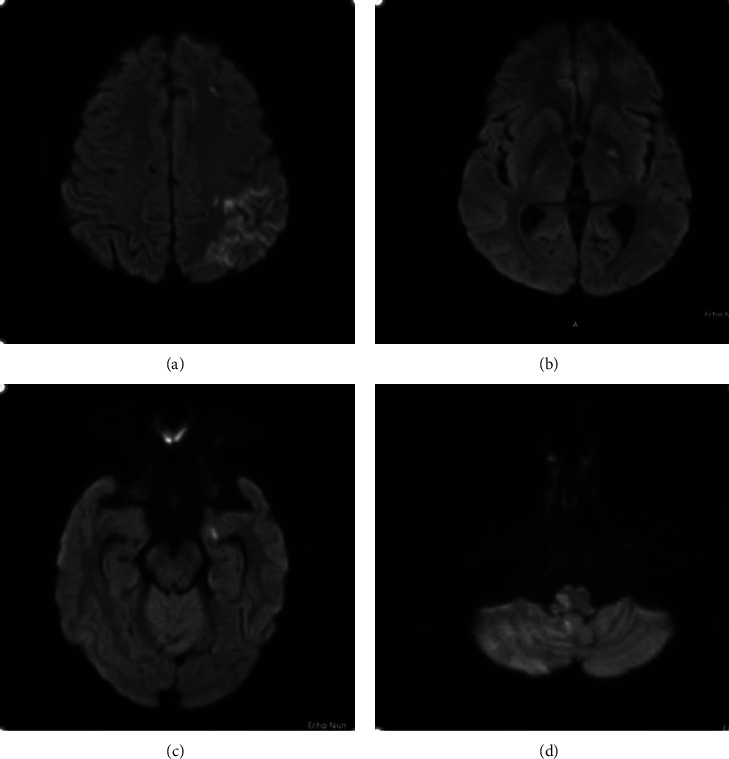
MRI head diffusion-weighted imaging showing diffusion restriction in the following areas: (a) right frontal and parietal lobe, (b) right internal capsule, (c) right temporal lobe, and (d) left medulla.
